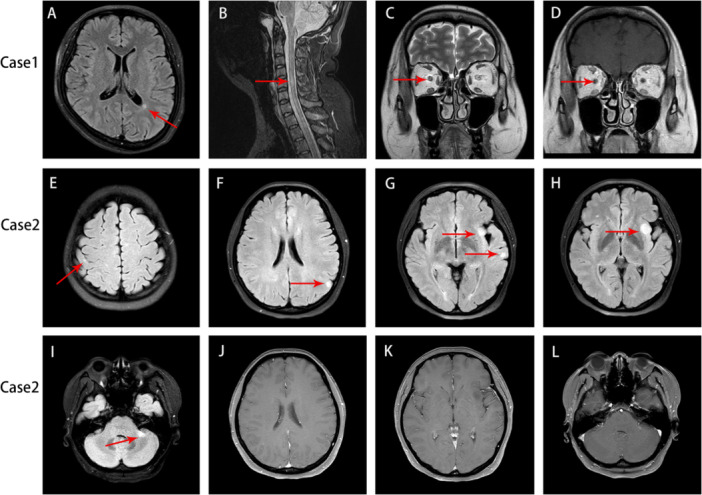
Case 1: Axial fluid‐attenuated inversion recovery (FLAIR) MRI sections revealing hyperintense lesions in the left periventricular regions and cervical segment (A and B). The orbital MRI showed anomalous T2 signals in both optic nerves, with a particular emphasis on the left eye (C and D). Case 2: Cranial MRI revealed abnormally hyperintense signals on FLAIR sequences in the left parietal lobe, left insula, temporal lobe, pontine brachium, left pons, and right frontal lobe (E–I) and no significant enhancement was observed (J–L).
